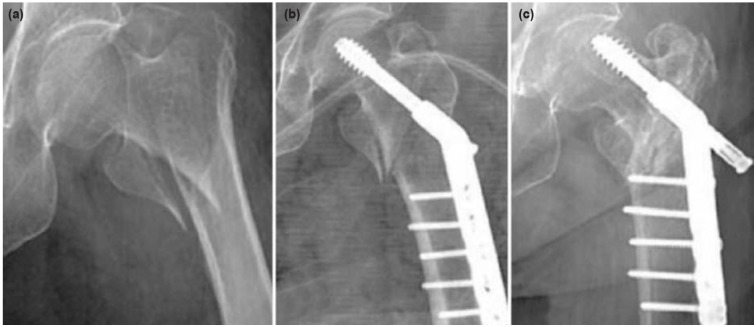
(a) Anteroposterior pre-operative, (b) Post-operative and (c) Final follow-up radiographs showing an unstable intertrochanteric fracture fixed with DHS.
